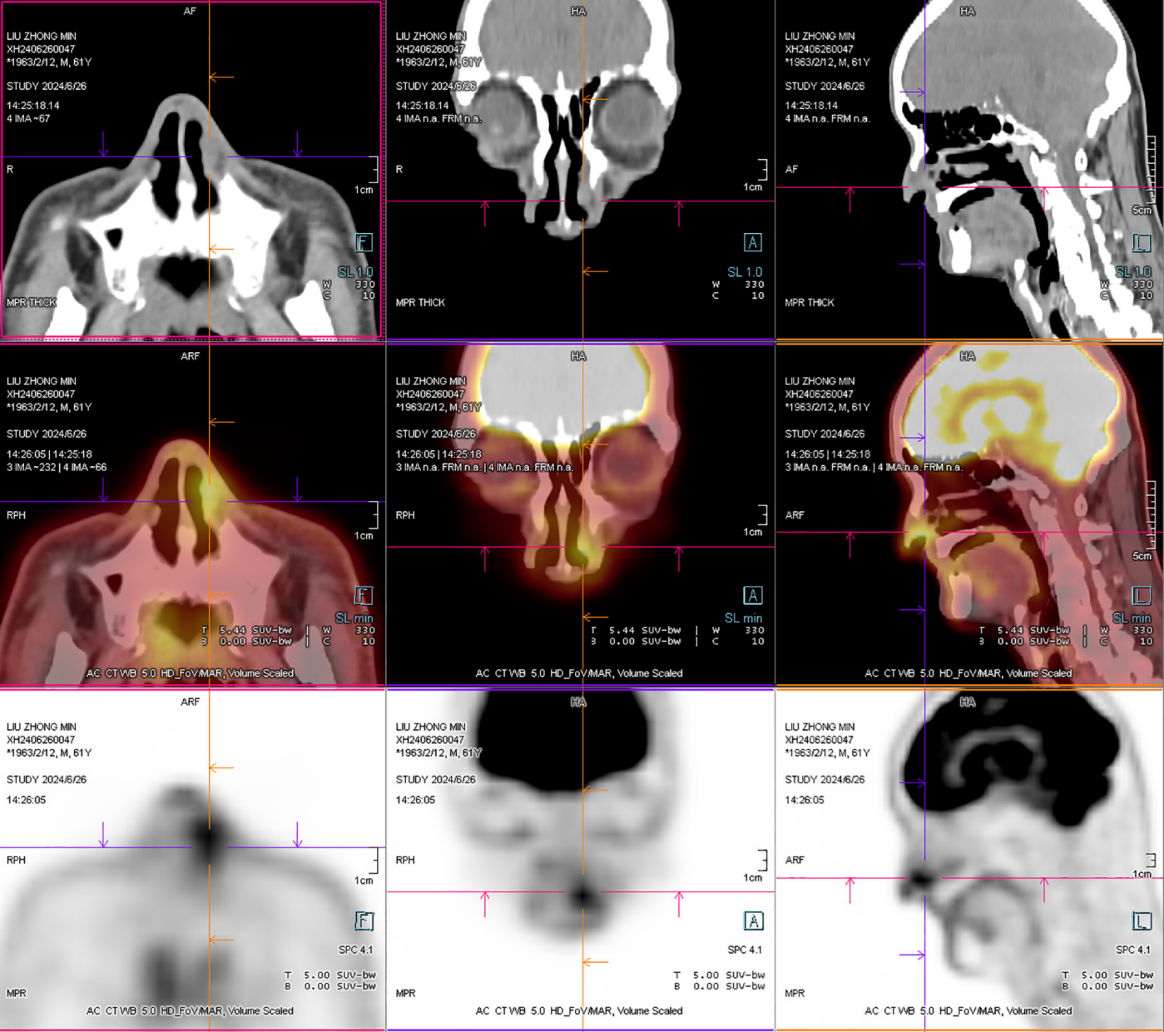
After operation PET-CT show that the soft tissue shadow of the left nasal cavity and the anterior part of the nasal septum is slightly thickened, and the FDG metabolism is increased, which may be consistent with lymphoma (open arrows). No lymphoma metastasis was seen. (The PETCT whole-body scan examination revealed no FDG hypermetabolic signals in other parts except for the lesion in the nasal cavity. Therefore, metastasis in other parts can be excluded.
